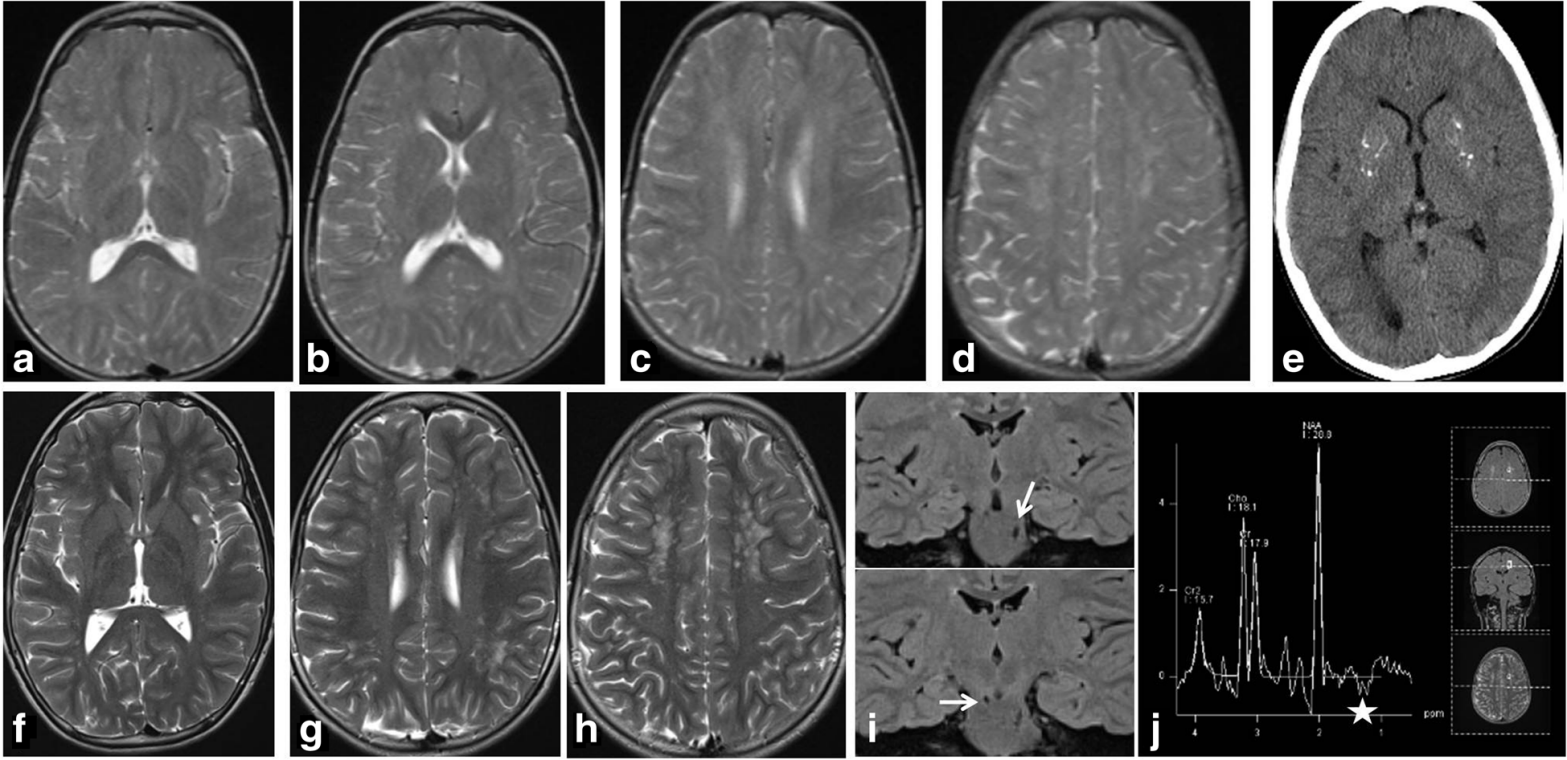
Atypical AGS – Top, brain MRI at 4 years old, (a-d), demonstrate mildly hyperintense T2 signal of the white matter relative to the cortex probably due to delayed myelination; CT show (e) basal ganglia calcification. Bottom, (f-j), brain MRI at 11 years old demonstrate myelination progression and multiple small confluent T2 hyperintensities in the sub-cortical areas mainly in F1. Enlarged perivascular spaces are visible in the brainstem (arrows in i); MRS in j shows lactate (asterisk) in the abnormal frontal white matter
